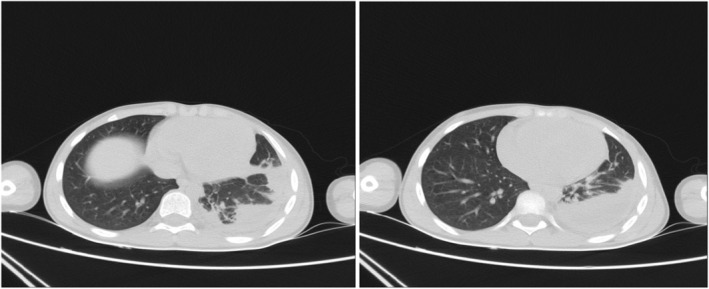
Spiral chest CT scan showing a left chest wall mass containing air bubbles and thickened septa in favor of empyema.
